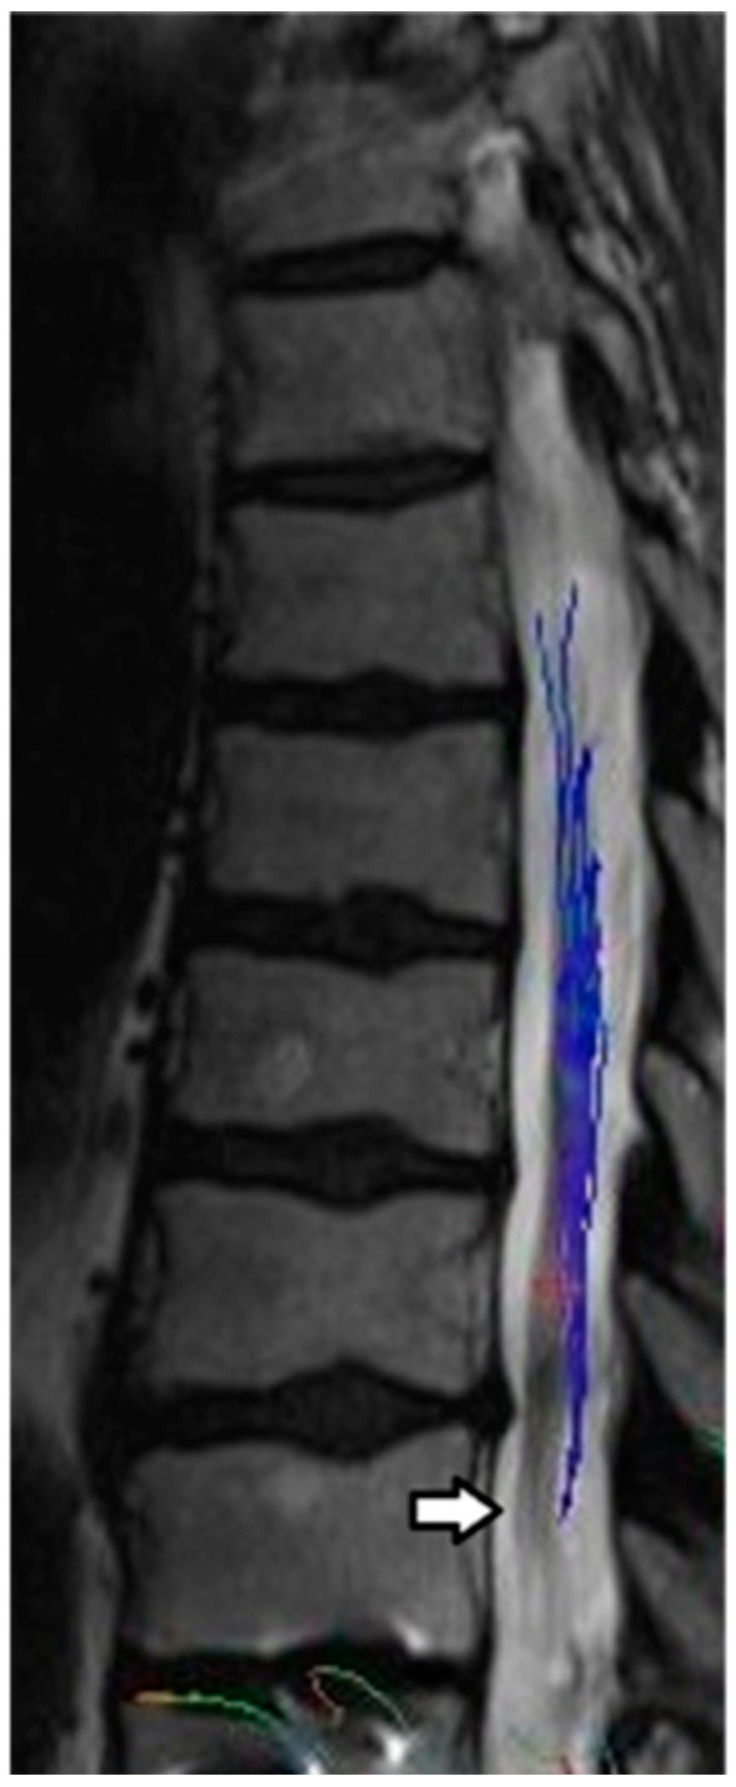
Sagittal T12 post processing MRI diffusion tractography imaging (DTI) demonstrates tapering of tract fibers in the lower thoracic cord proximal to the level of injury, corresponding to the volume loss, in keeping with Wallerian degeneration.
